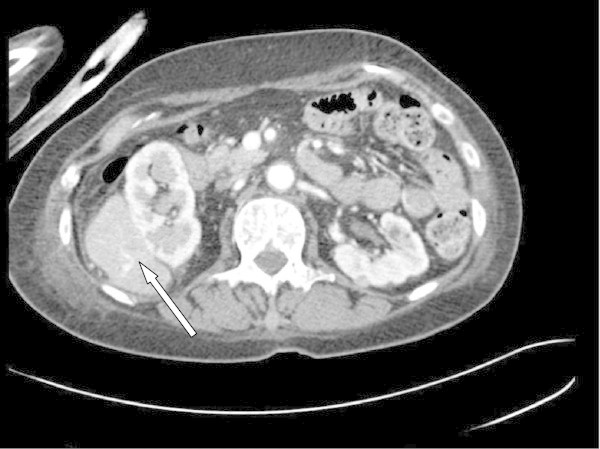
Initial CT showing retroperitoneal mass compressing on the right kidney.
